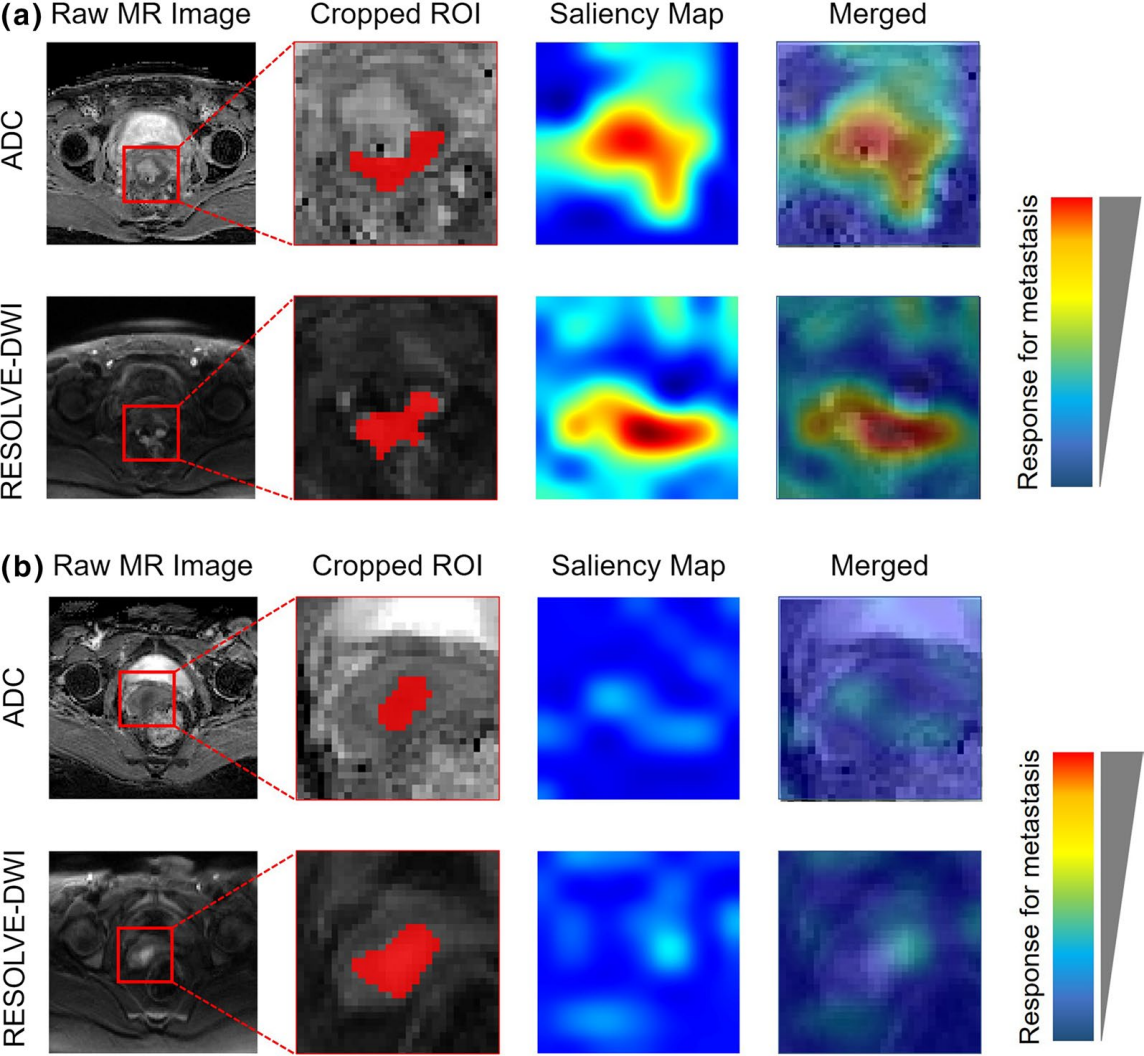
Saliency maps for the combined model. The ADC and RESOLVE-DWI modalities of the MR images and the corresponding saliency map of a metastasis example (a) and a non-metastasis example (b) were shown, respectively. The red color highlighted the activation region associated with the prediction of metastasis or non-metastasis
